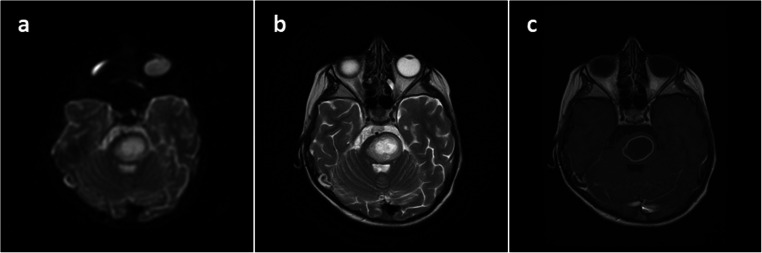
Axial cranial MRI a DWI, b T2-weighted, and c T1-weighted without gadolinium showing pontine abscess
